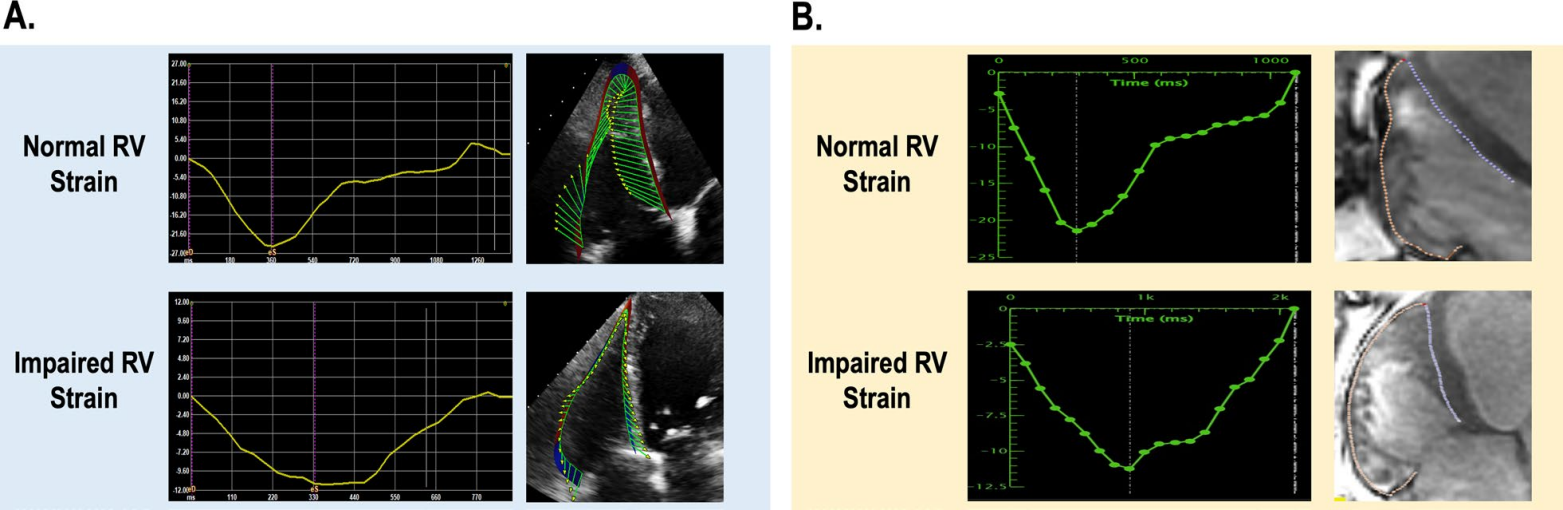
a Representative example of RV strain as quantified using echocardiography. Note differential peak strain characteristic of normal RV GLS (top) and impaired RV GLS (bottom). b Representative example of RV strain as quantified using CMR. Note differential peak strain characteristic of normal RV GLS (top) and impaired RV GLS (bottom)
